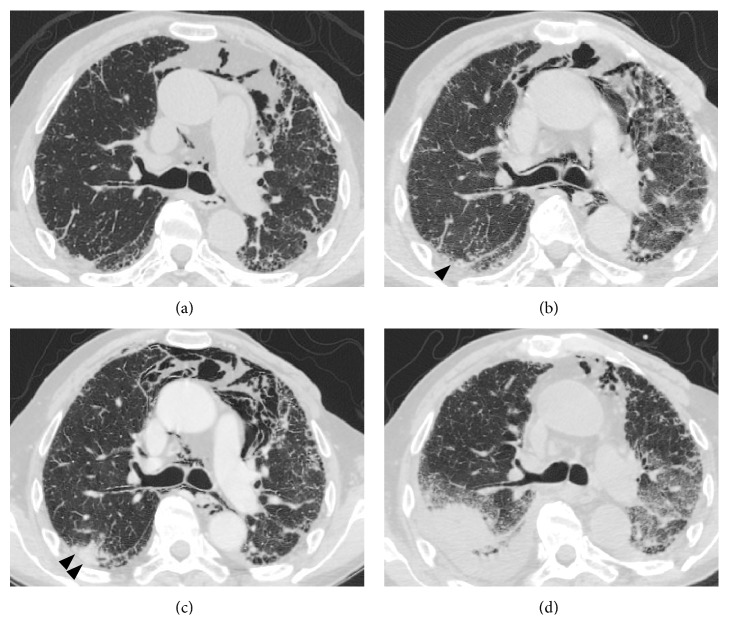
Serial changes in chest computed tomography findings. (a) Computed tomography (CT) scan taken 3 months before admission, showing reticular opacities and small cystic airspaces predominantly in subpleural regions. Mediastinal emphysema is also visible. (b) CT scan taken at admission, showing minimal mediastinal emphysema without major signs of pneumonia. Small centrilobular nodules are visible in the right upper lobe (arrowhead). (c) CT scan taken on the 20th day of hospitalization, showing consolidation in the right upper lobe (arrowheads). (d) CT scan taken on the 77th day of hospitalization, showing increased consolidation size with emergence of diffuse ground-glass opacities in both lungs.
